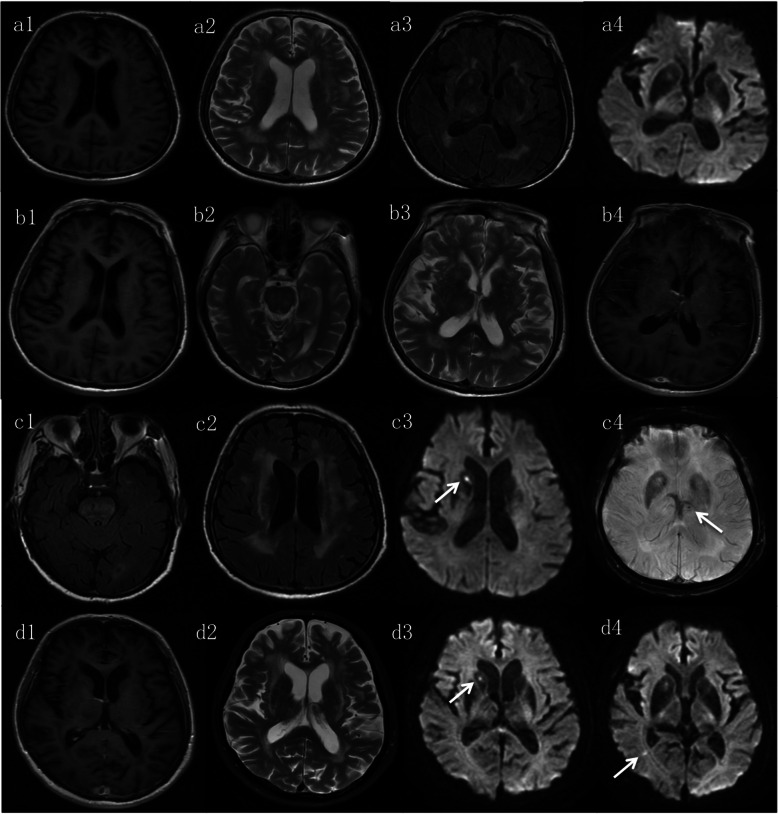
MRI findings of the patient. MRI on May 31, 2019 (a1–a4) showed patchy abnormal lesions in the bilateral thalamus, basal ganglia, pons, and bilateral white matter on T2 and FLAIR image. MRI with contrast on June 5 (b1–b4) revealed no enhanced lesions on the T1 image and the abnormal lesions on the T2 image were similar to the previous MRI scan. On July 26 (c1–c4), the FLAIR images revealed that abnormal signals were more severe and diffused than the previous MRI findings. DWI image showed new cerebral infarction in the right basal ganglia, and SWI found a small hemorrhage in the left thalamus (indicated by arrows). MRI with contrast on July 30 (d1–d4), new cerebral infarction was found in the right optic radiation, and the infarction of the right basal ganglia was similar to the previous scan (indicated by arrows). Also, no lesions were enhanced. DWI, diffusion-weighted imaging; FLAIR, fluid-attenuated inversion recovery; MRI, magnetic resonance imaging; SWI, susceptibility-weighted imaging
